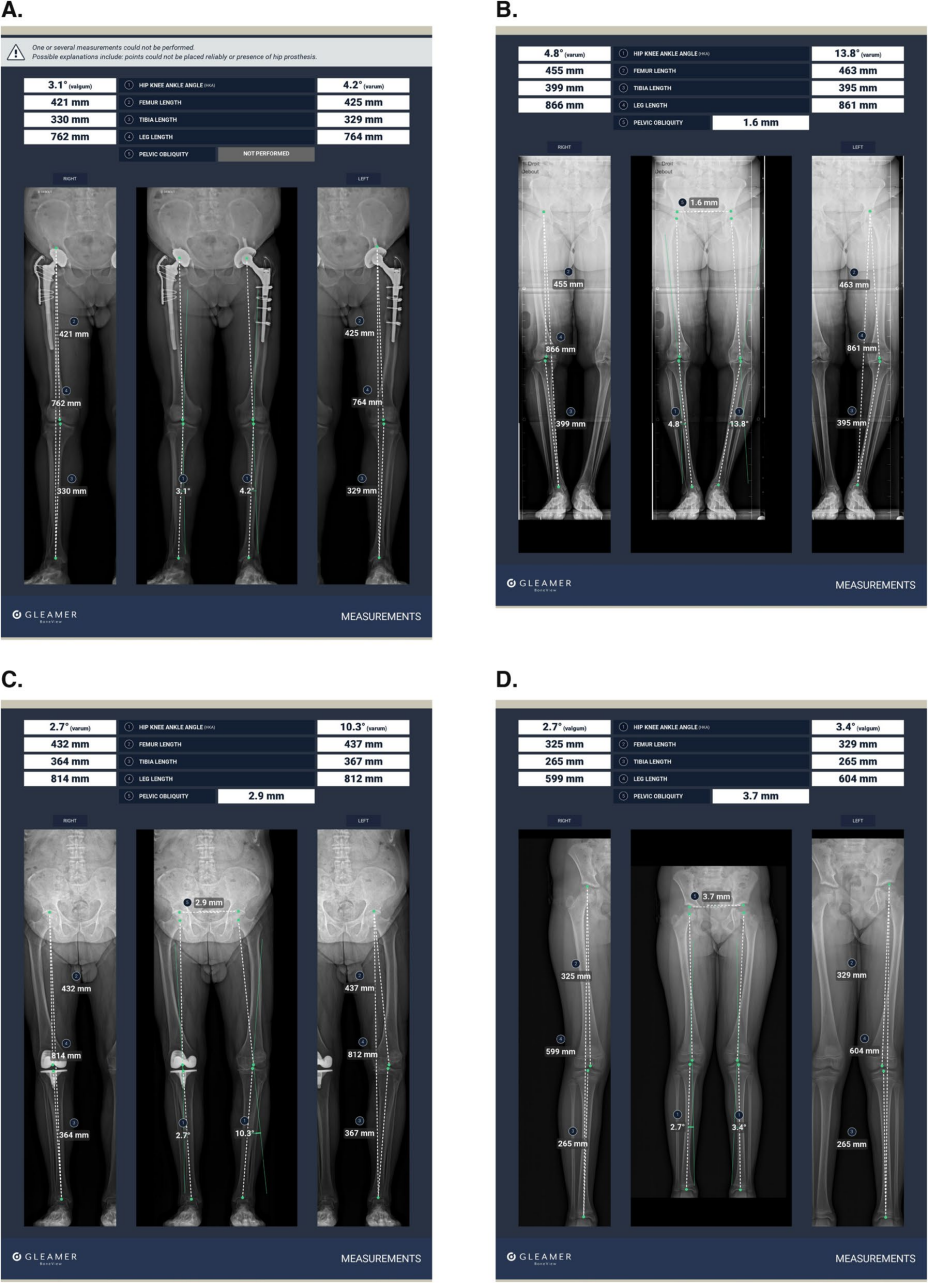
Examples of radiographs processed by the AI algorithm. A A 60-year-old male patient with bilateral hip prostheses. Note that pelvic obliquity is not processed by the AI algorithm if the patient has a hip prosthesis. B A 73-year-old female patient with bilateral genu varum deformity. C A 78-year-old male patient with a right knee prosthesis and severe left genu varum deformity. D An 8-year-old girl with genu valgum deformity
